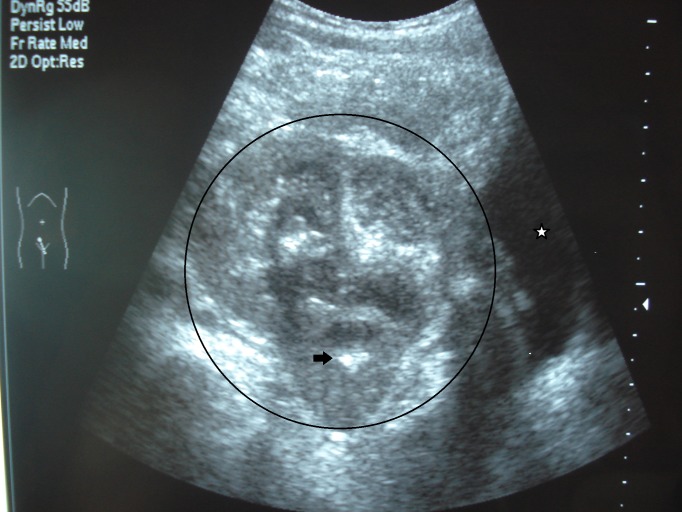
Ultrasound of the pelvic in axial section showing a heterogeneous mass (black circle) and calcification (black arrow) in close proximity to the urinary bladder (asterisk).
